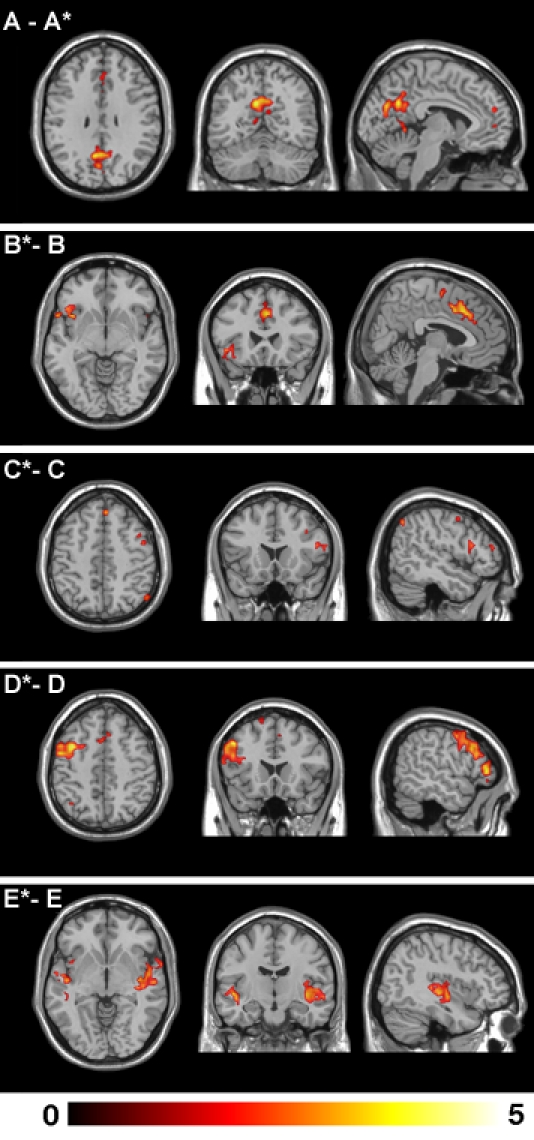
Modulation of Resting-State Networks by Mood Induction. Global functional connectivity differences observed between the neutral and sad recall conditions for the common RSNs. The top panel A - A* indicates an apparent *decrease* in functional connectivity of the default mode network between the neutral and sad recall conditions. All other panels indicate apparent *increases* of functional connectivity during the sad compared to neutral recall condition. All images are presented on a high-resolution single-subject MRI in standard neuroanatomical space (Montreal Neurological Institute, Colin-27). Corresponding color bars indicate the t score ranges of the displayed maps. Images are displayed in neurological convention (left = left).
